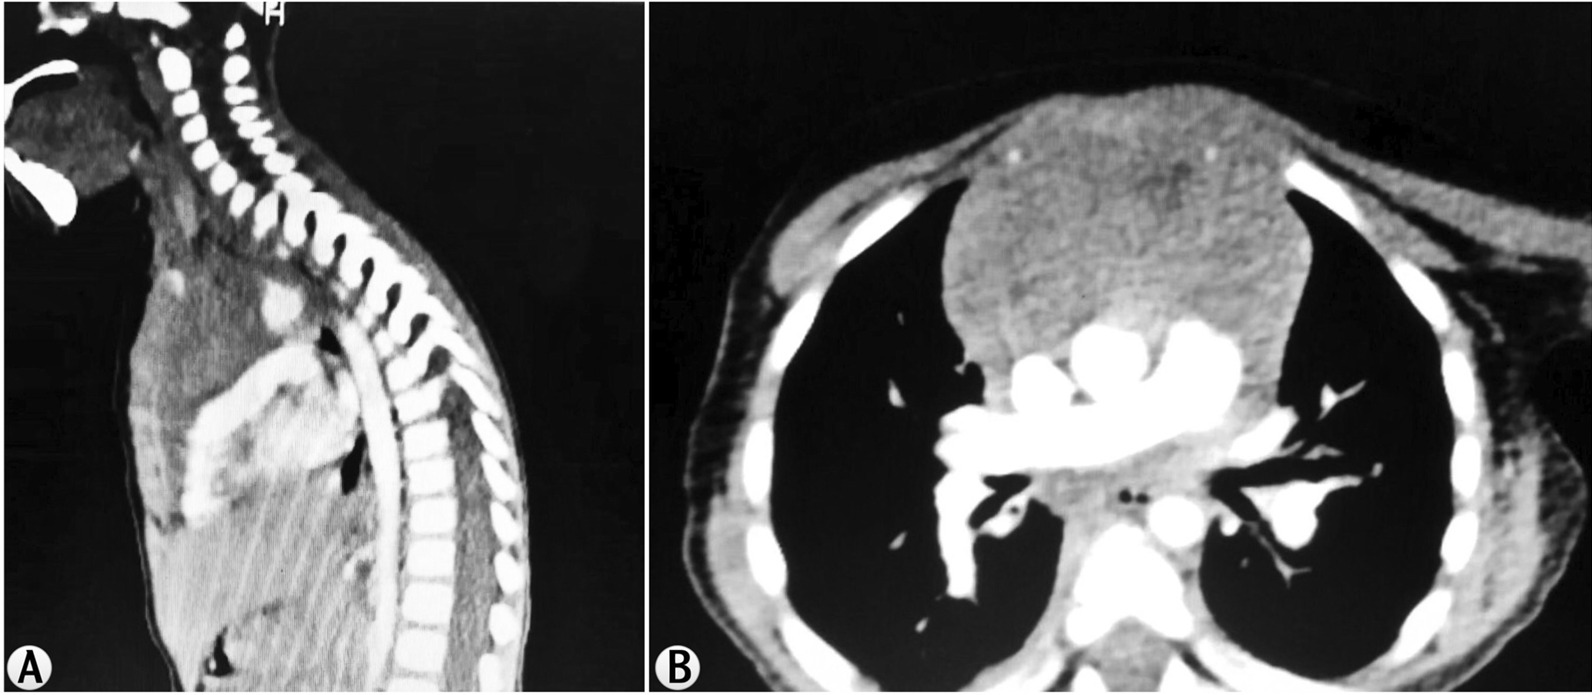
CT findings in case 2. The axial (a) and sagittal (b) CT planes show a large mediastinal mass rounding the neck vessels measuring 53 × 38 mm, extending to the thoracic cave around the branching of bronchi measuring 30 × 29 mm compressing both bronchi
